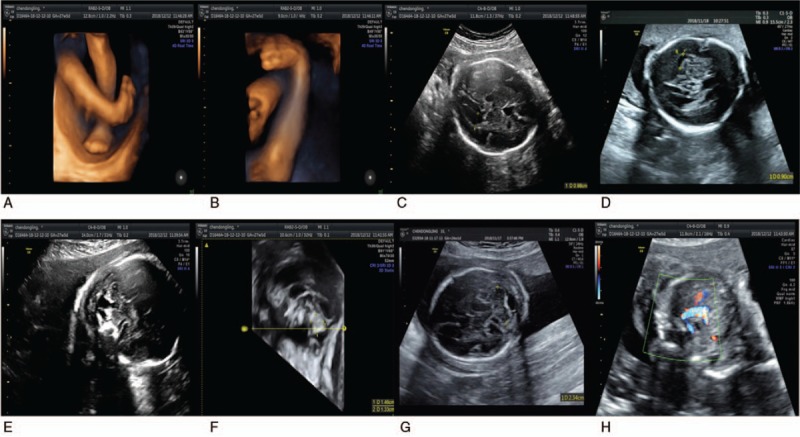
Fetal ultrasound A, B: Right clubfoot; C, D: abnormally enlarged brain ventricles: left 0.98 cm, right 0.90 cm; E, F, G: an enlarged cisterna magna: 0.65 cm, anterior-posterior length of the cerebellar vermis, height of the cerebellar vermis, transcerebellar diameter; H: aberrant right subclavicular artery, mild tricuspid reflux.
